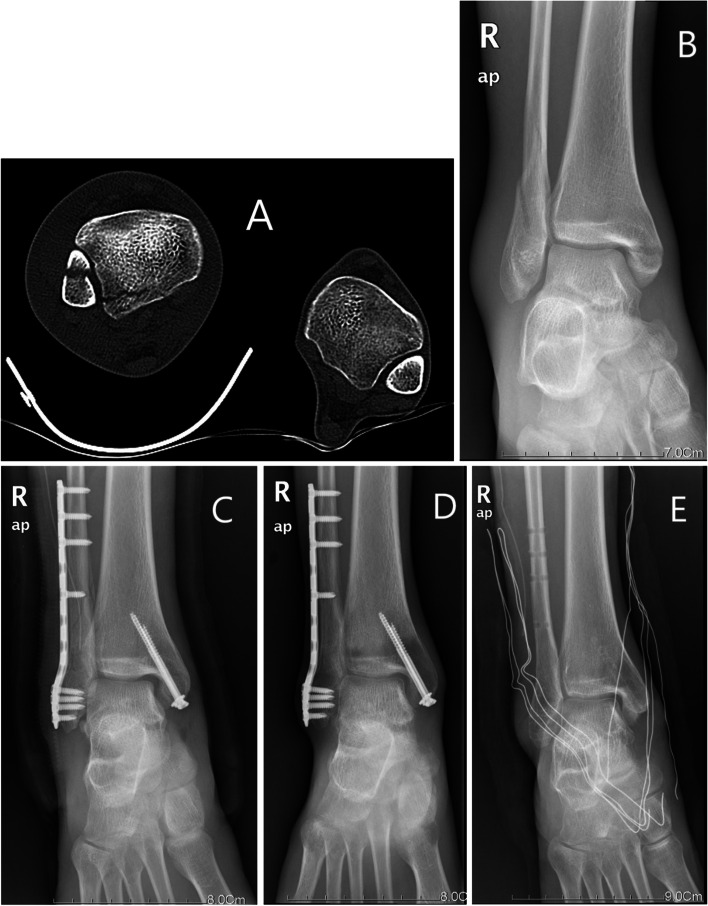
CT and X-ray images of AITFL anatomical repair. Notes: This patient underwent the AITFL anatomical repair technique. The radiolucent anchor and rope were not showed in the radiograph. A Preoperative CT image. B Preoperative radiographs image. C Radiograph at 1 days postoperatively. D Radiograph at 3 months postoperatively. Postoperative radiographs showed ankle fracture reduction maintained well. E Radiograph after removal of internal fixation
